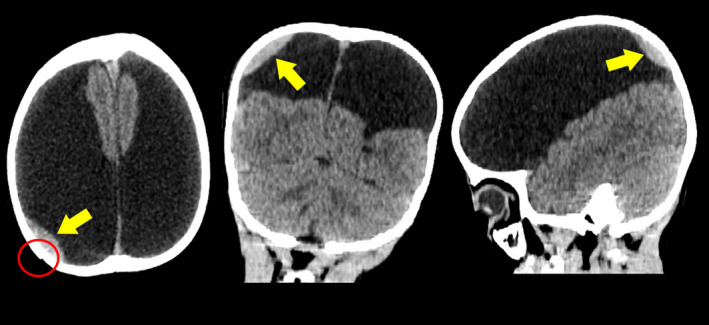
Axial, coronal, and sagittal CT images show skull bone fracture (red circle) and epidural hematoma (yellow arrows) in the right parietal region
